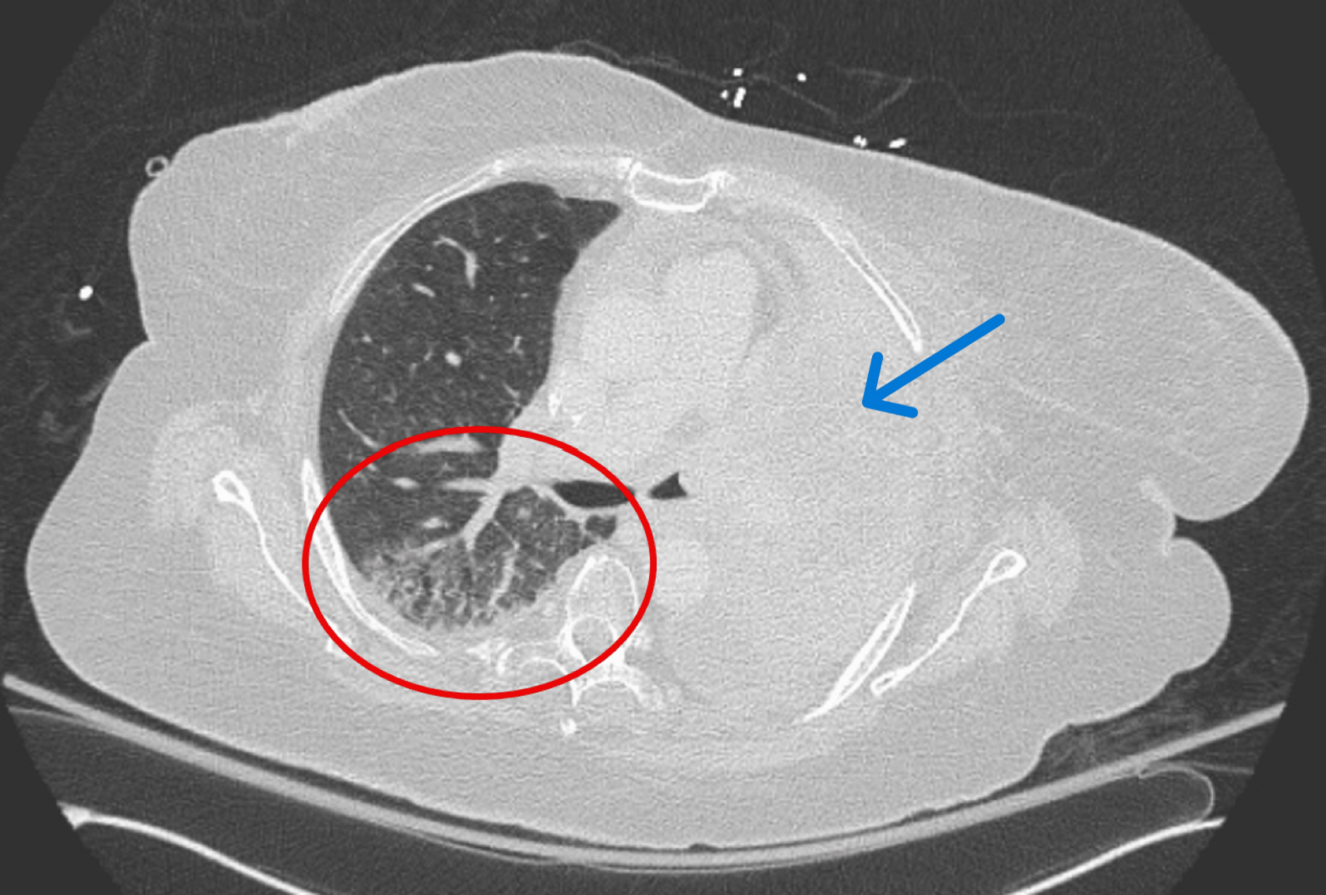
CT Chest on Day 9 of Hospitalization Ground-glass opacities throughout the right lung, most prominent posteriorly (red circle). Stable left bronchus occlusion (blue arrow) with no new foci. These new findings support a diagnosis of checkpoint inhibitor pneumonitis, explaining the patient's worsening respiratory status despite empiric antibiotics, diuretics, and bronchodilators.
